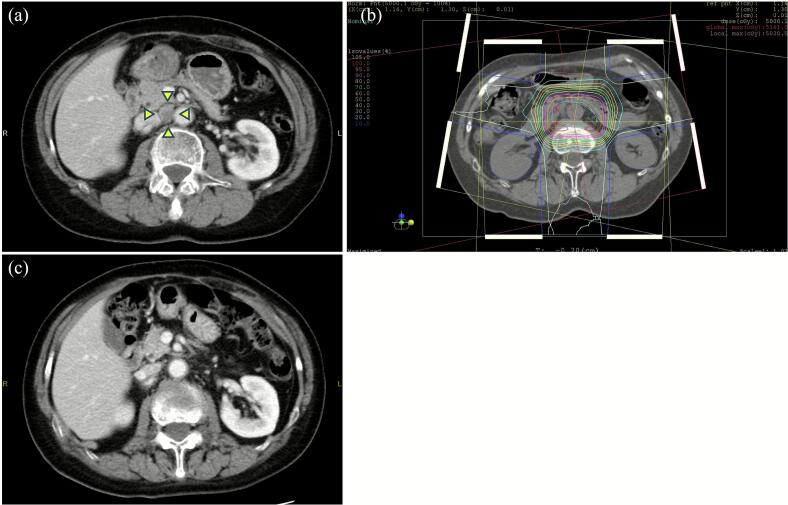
A representative case demonstrating the efficacy of proton beam therapy (PBT). This patient presented with a paraaortic lymph node metastasis that was treated with 66 Gy (RBE) using PBT. Fifty-eight months after treatment, this lesion remains in complete remission. (a) CT image showing the metastatic paraaortic lymph node before PBT (arrowheads). (b) Dose distribution of initial PBT treatment plan. (c) CT image obtained 2 months after completion of PBT. RBE, relative biological effectiveness; CT, computed tomography; PBT, proton beam therapy.
